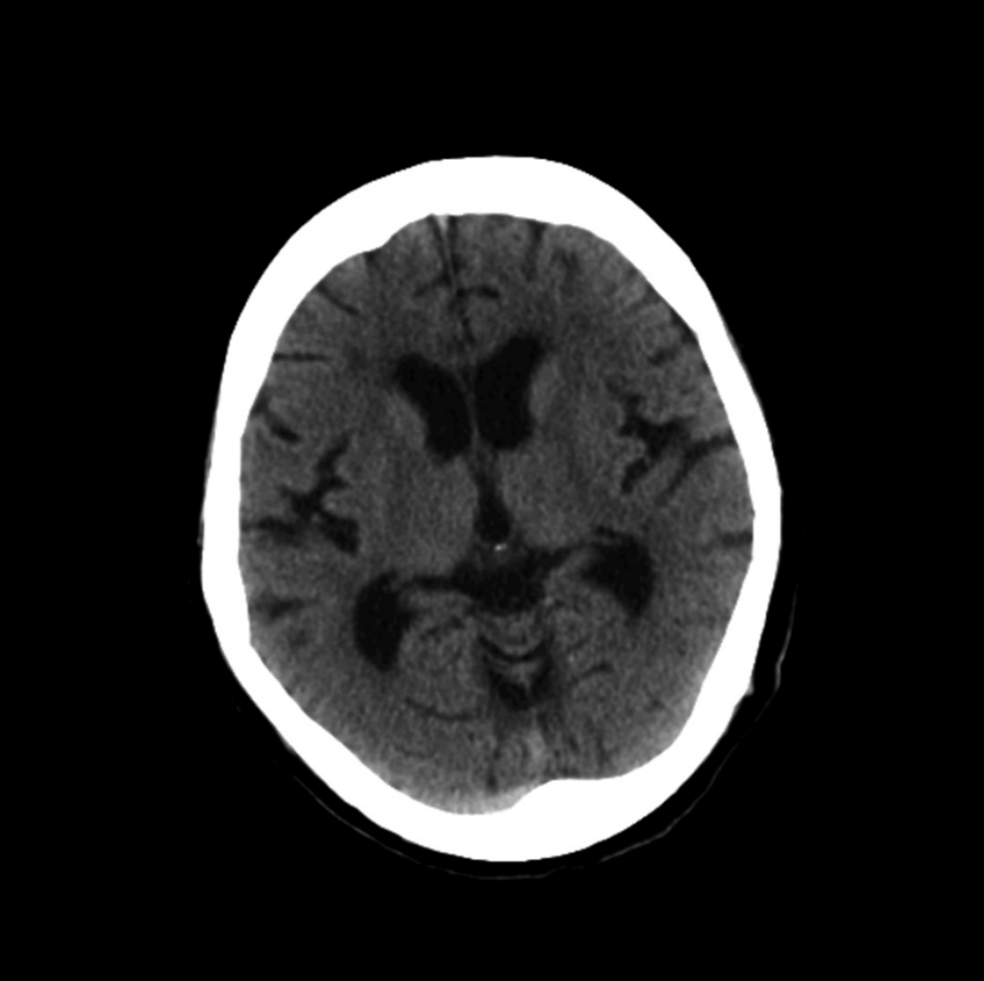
Plain CT of the head There was no abnormality.
